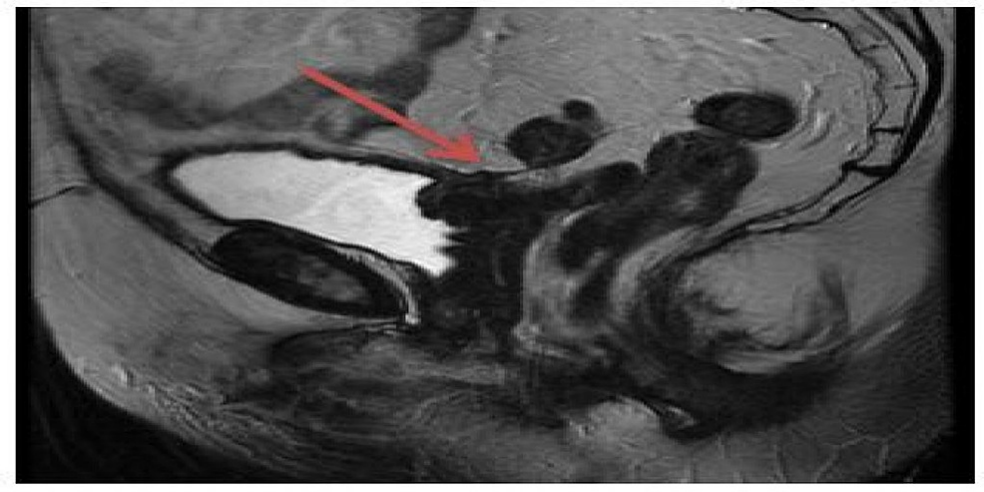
MRI-pelvis: sagittal view (T2 fast relaxation fast spin-echo sequence). Linear area of soft tissue tethering the distal sigmoid colon to a thickened area of the posterosuperior urinary bladder wall. These features likely represent a colovesical fistula.
